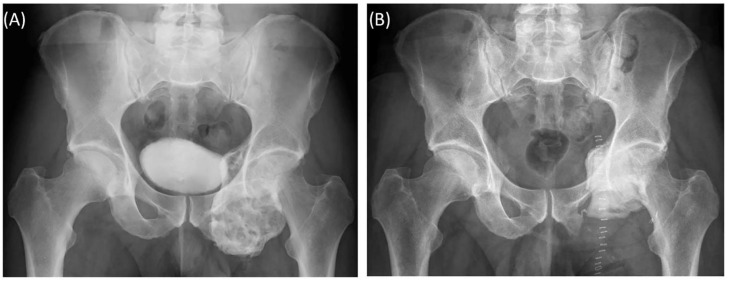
Case 5: Preoperative plain radiograph of the acetabular giant cell tumor (A) and postoperative result after resection of the dorsal parts and intralesional curettage with a navigated burr and augmentation with polymethyl methacrylate (B).
